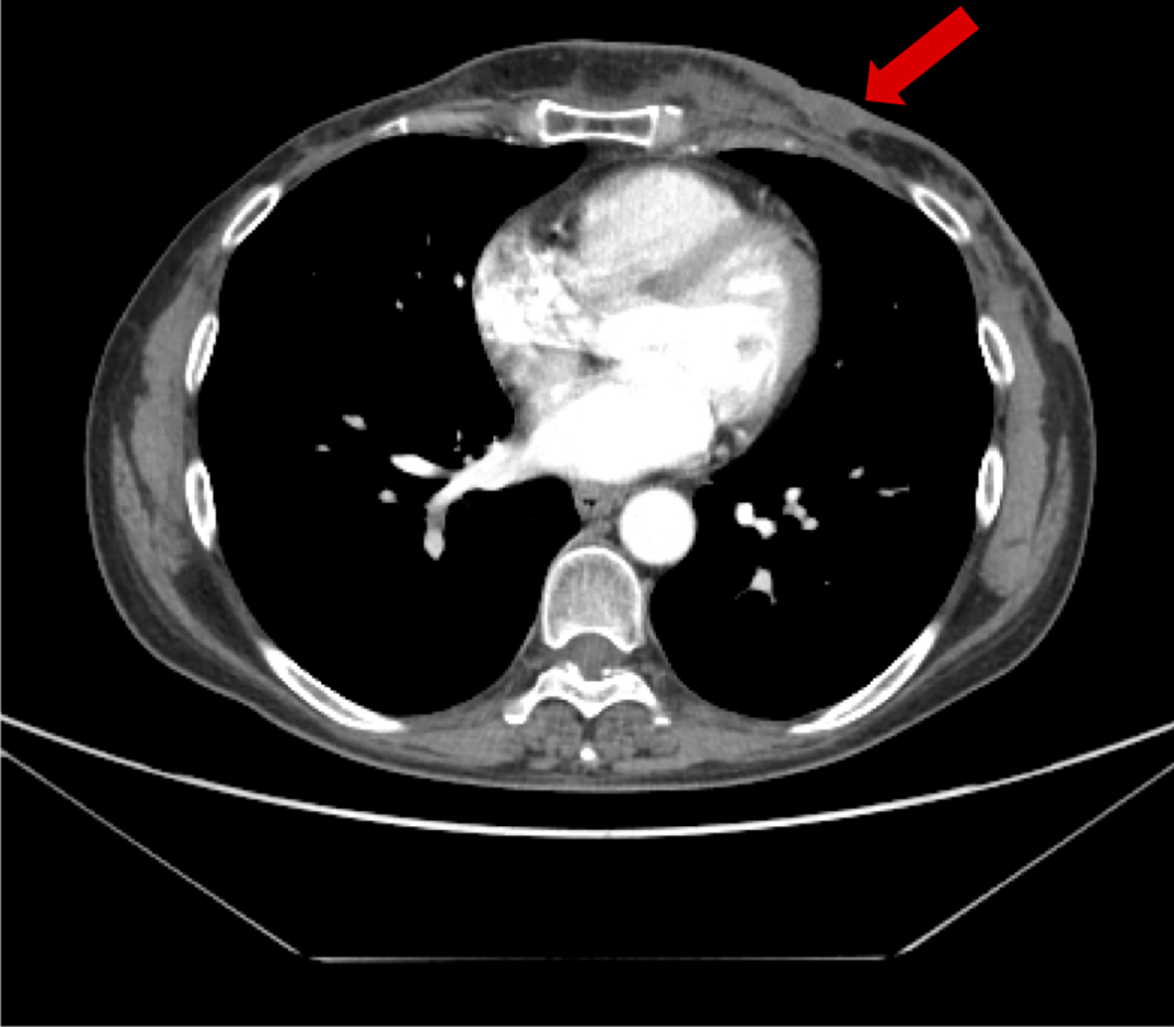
First evidence of metastatic disease on baseline PET-CT scan.
